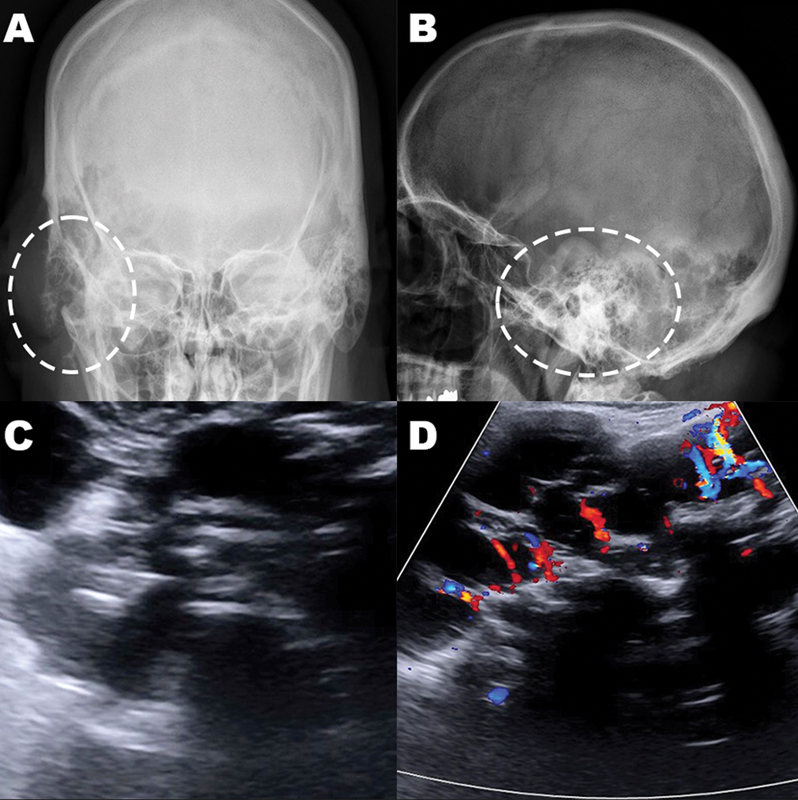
Skull x-ray anteroposterior (
